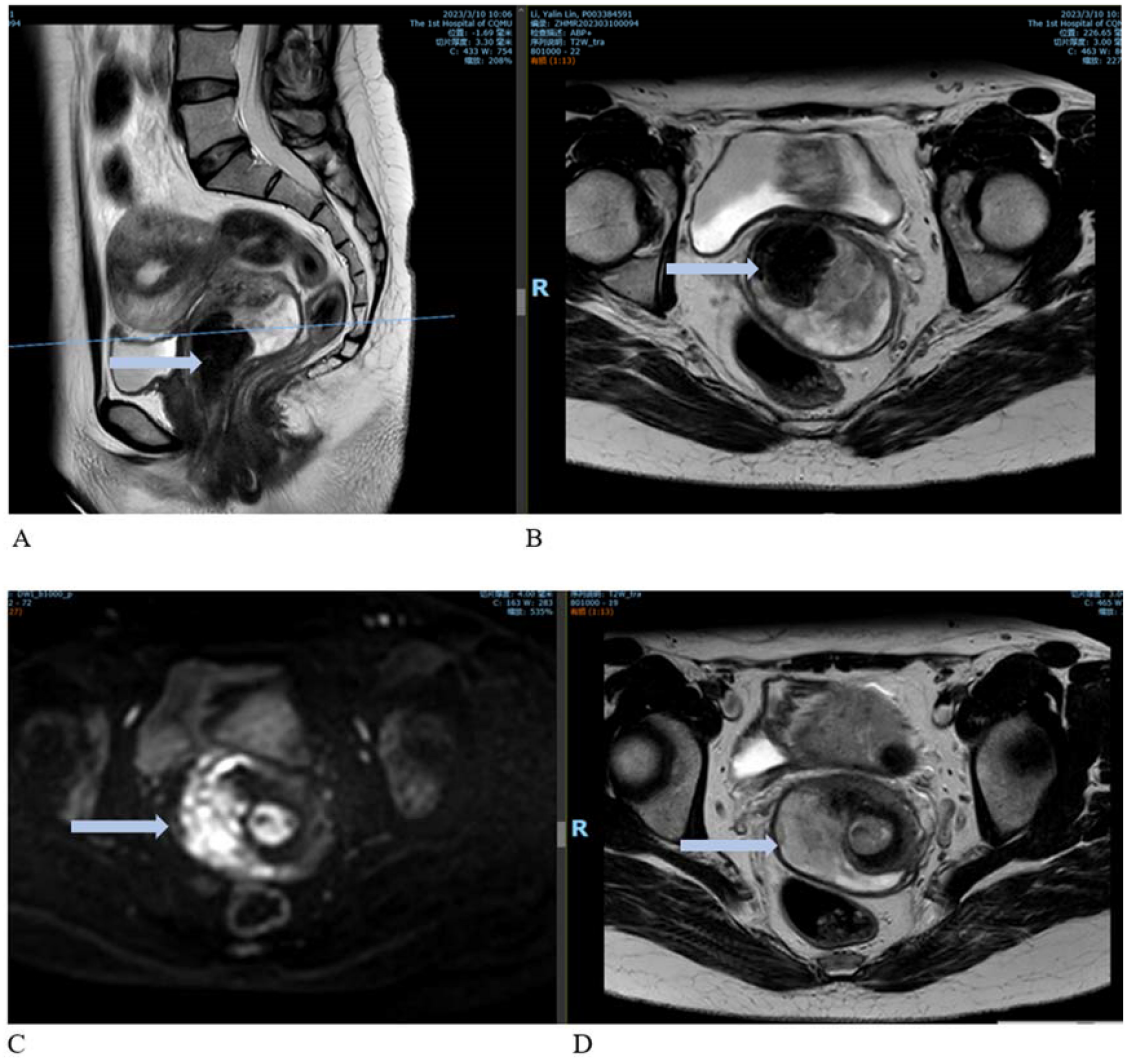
Contrast-enhanced MRI images. (A) Sagittal view showing an enlarged cervix with an irregularly shaped mass extending to the internal cervical orifice and into the vagina. (B) Cross-sectional view of the lesion. (C, D) Enhanced images showing marked enhancement at the cervical lesion site.
